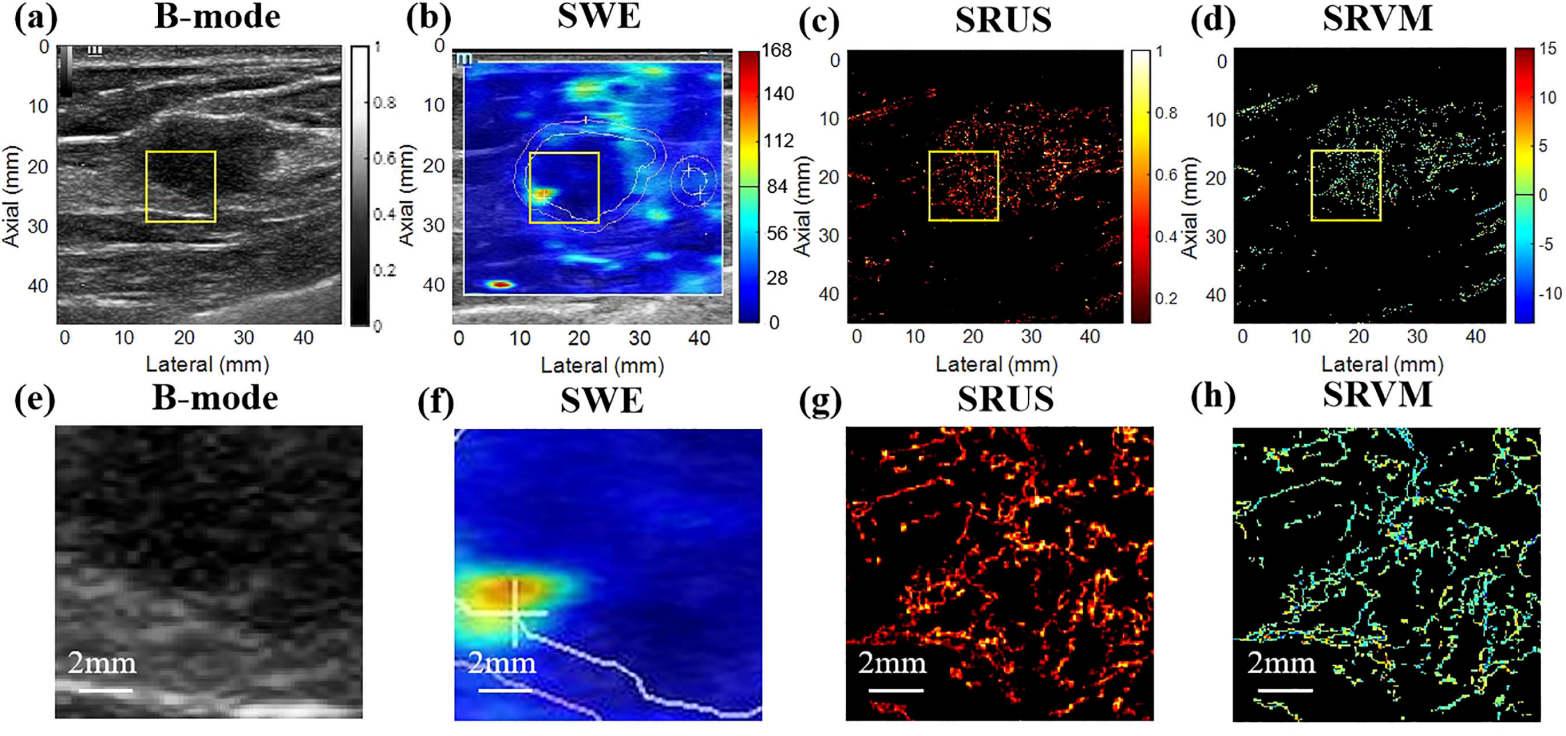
Ultrasound images of malignant breast masses. (A) B-mode; (B) shear-wave elastography; (C) super-resolution ultrasound imaging; (D) super-resolution velocity map. (E–H) were the details of the breast mass between (A) B-mode, (B) shear-wave elastography, (C) super-resolution ultrasound imaging, and (D) super-resolution velocity map, as shown in the yellow box in the figure.
